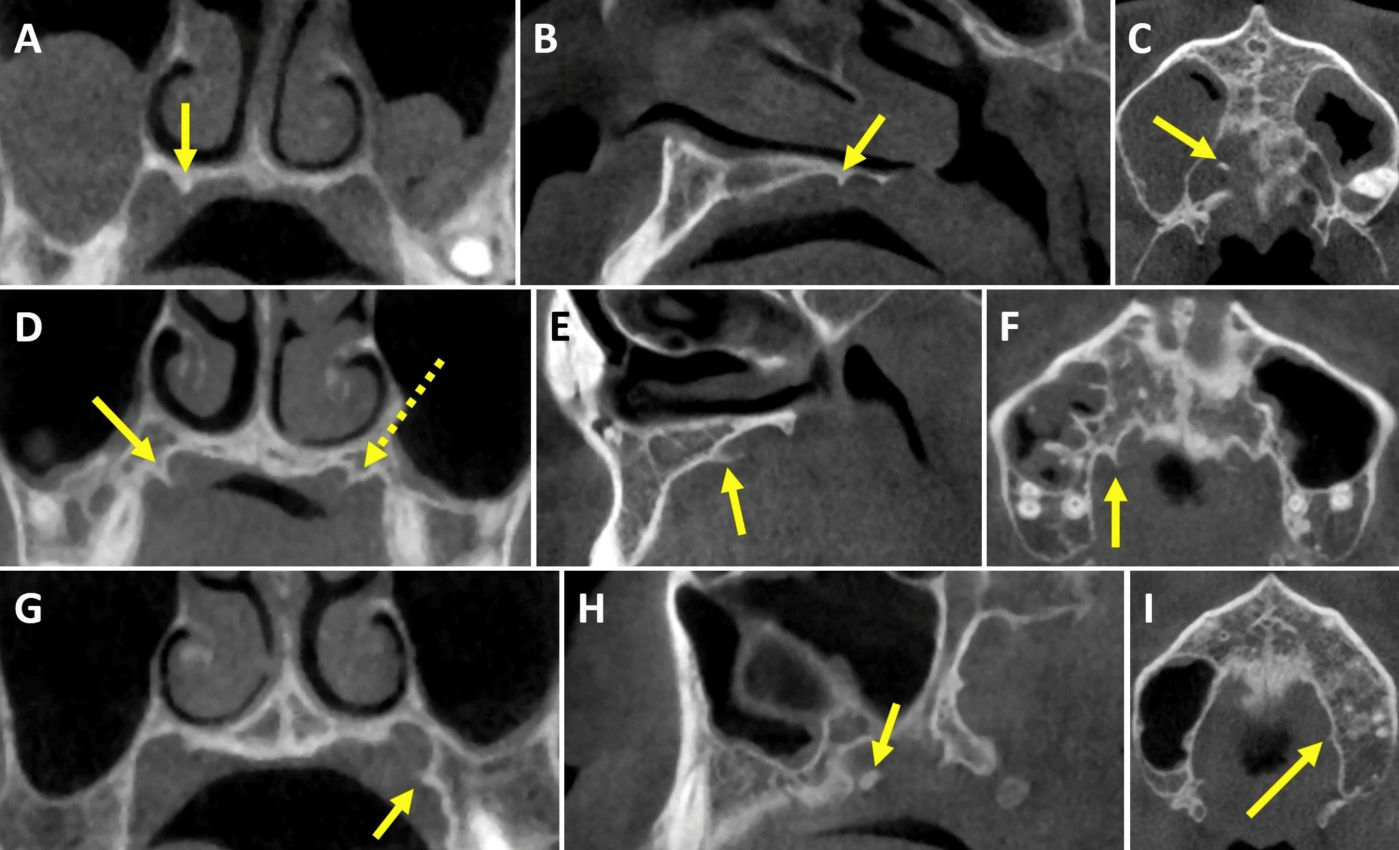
Multiplanar CT images showing the three locations for PSs Medial PSs are shown for the same patient in (A-C), middle PSs for a different patient in (D-F), and lateral PSs also for a different patient in (G-I). All arrows point to PSs in the coronal (A,D,G), sagittal (B,E,H), and axial planes (C,F,I). The dashed arrow in (D) shows a left middle PS in addition to the right middle PS (solid arrow). Coronal images were used for measurements of PSs and their mucosal coverage. Sagittal and axial images are shown here for clarification of PS anatomy and location. PS: Palatine spine
